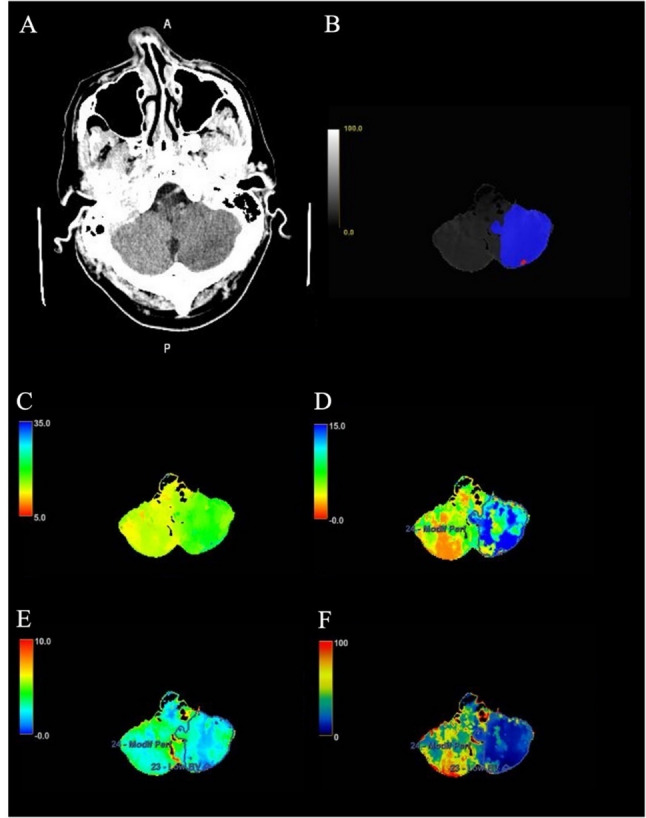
Initial CT and perfusion imaging: Axial NCCT demonstrating a hypodense infarct in the left cerebellar hemisphere A; tissue classification map B; Time to Peak map demonstrating prolonged perfusion within the infarct territory C; Mean Transit Time map showing delayed transit D; Cerebral Blood Volume map demonstrating reduced blood volume E; Cerebral Blood Flow map demonstrating reduced blood flow within the infarct territory F
